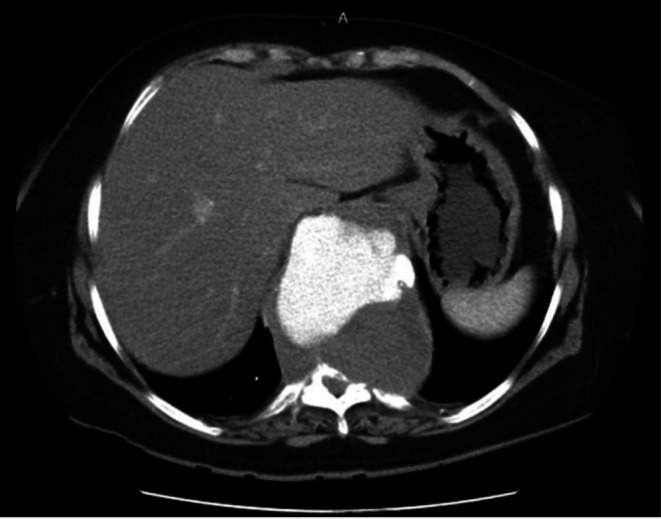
Preoperative axial CT angiography showing a large thoracoabdominal aortic aneurysm compressing adjacent thoracic structures. The aneurysm sac is visible at its maximum diameter (~7.0 cm), with early signs of surrounding vertebral body remodeling.
